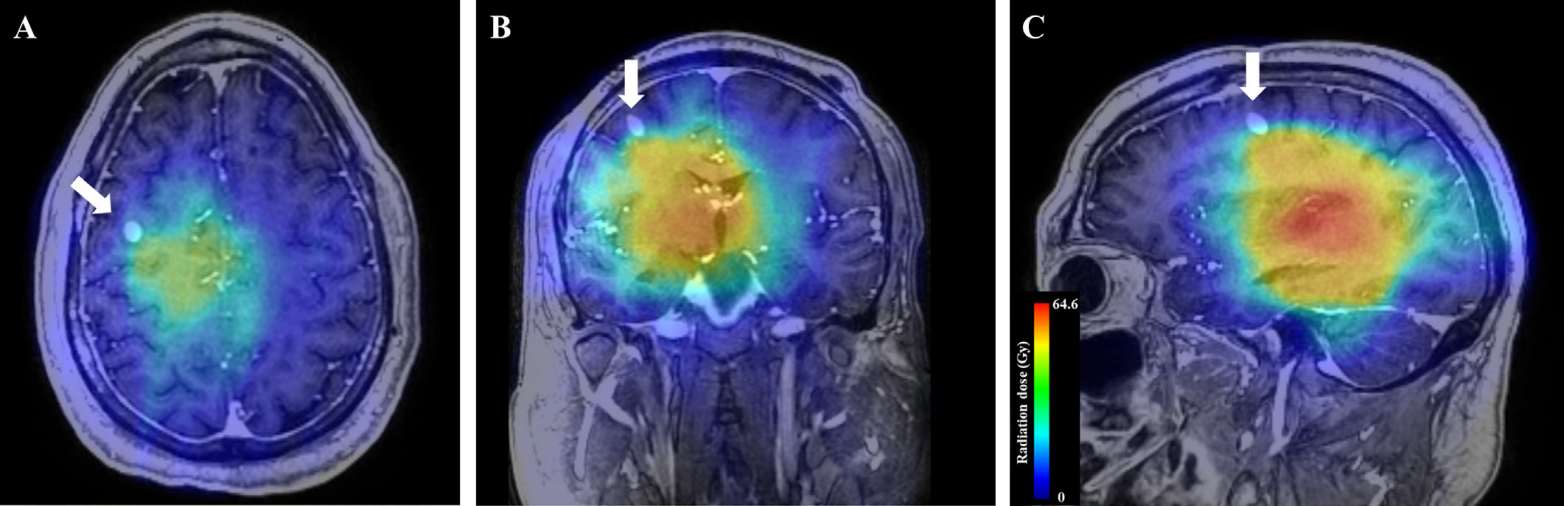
Radiation dose color maps superimposed on 3 month follow-up imaging. Dosing map superimposed on axial (A), coronal (B), and sagittal (C) T1W post-contrast MRI demonstrating the secondary lesion (white arrows). The distant nodule is outside the primary radiation field.
